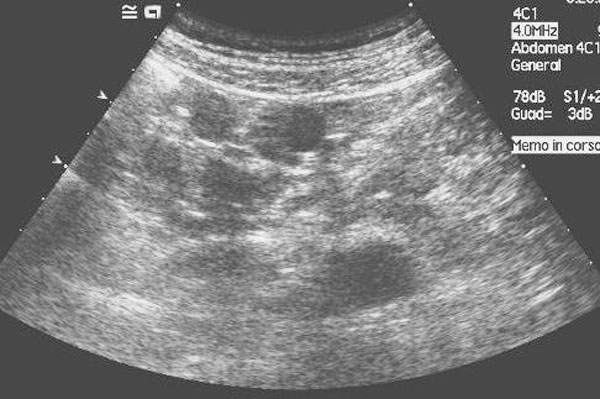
Second-look ultrasound of the nodule in figure 1. US shows homogeneous splenic-like echogenicity of the nodule in the paracolic space, thus confirming its splenic origin.
